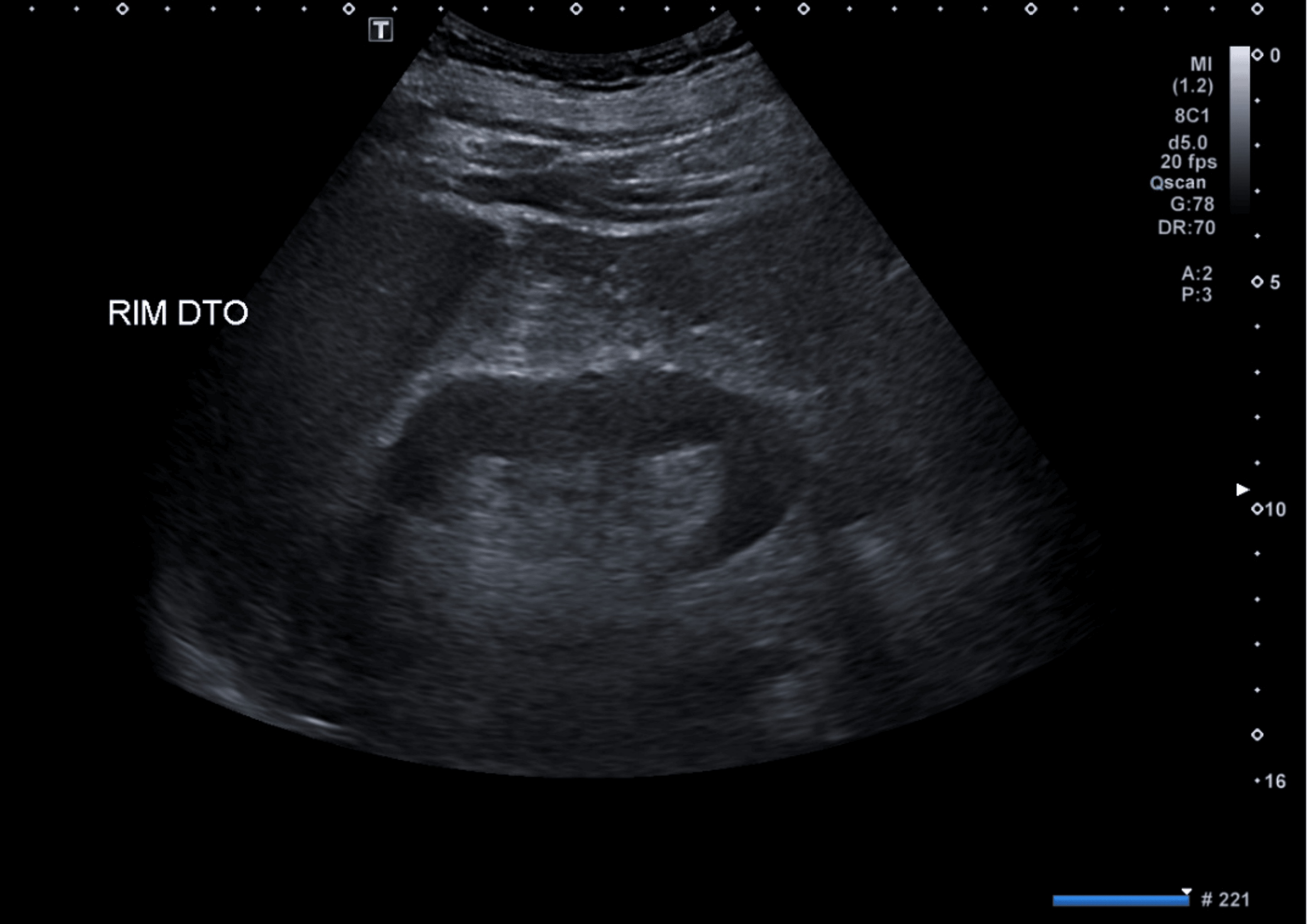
Right kidney ultrasound The kidneys were normal-sized and without morphological changes, showing normal parenchymal-sinus differentiation and no dilation of the renal excretory cavities on the right kidney.
